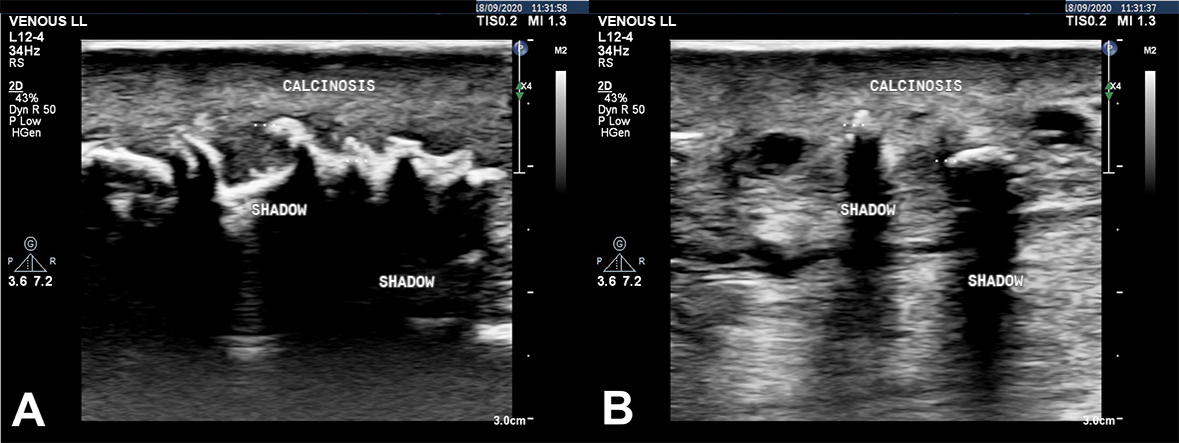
(A) and (B) Color Doppler ultrasound of the lower limbs (B-mode) with hyperechogenic images that generate intense acoustic shadows, with areas of convergence, suggestive of calcium deposits in the subcutaneous cellular tissues, in the mid-distal third of the leg in the region of the ulcers.
